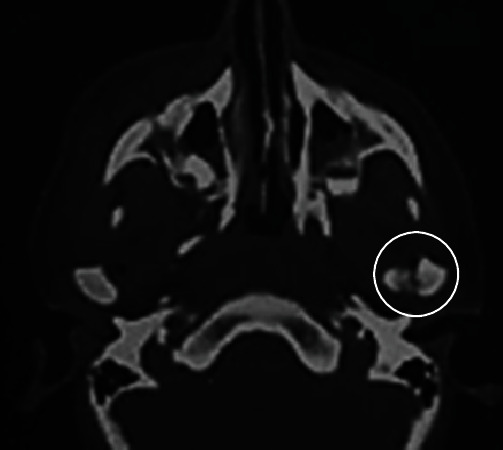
Immediate posttraumatic computed tomography scan. Axial slice in bone rendering showing fracture of the medial pole of the left condyle (white circle).
